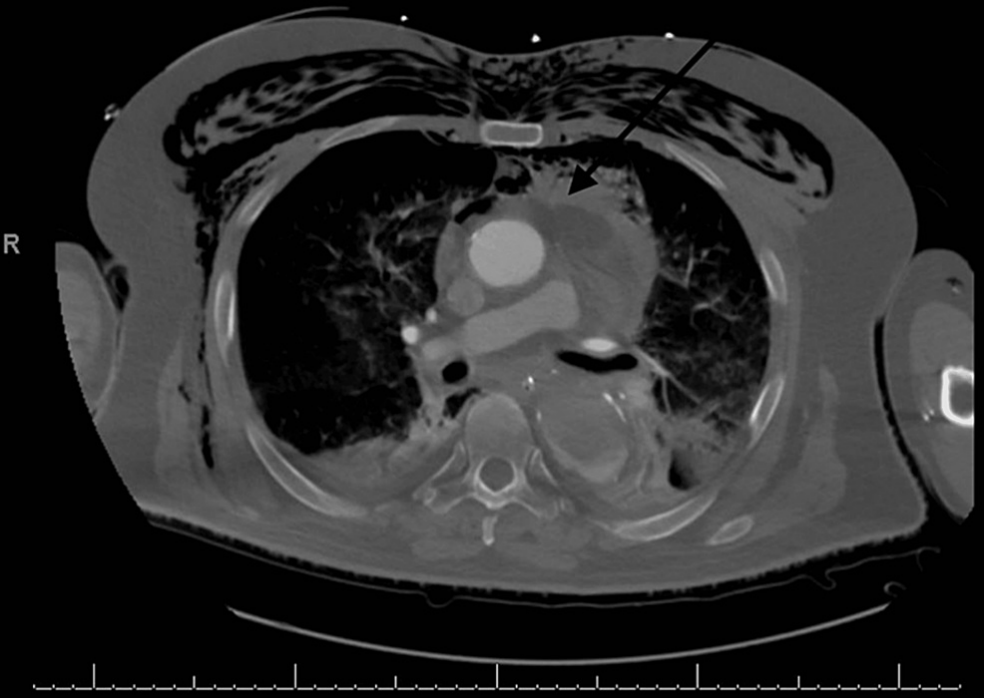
CT scan of the chest axial view showing hemopericardium; indicative of a potential aortic rupture (see black arrow)
